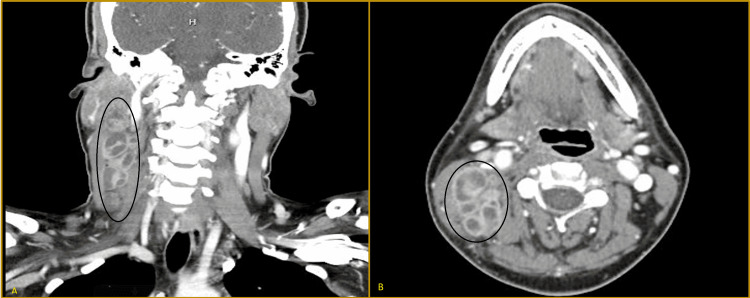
CT scan of the neck showing hypoattenuating/centrally necrotic glomerate lymph node mass (4.2 x 7.4 cm); Black circle in coronal section (A), and transverse section (B)
